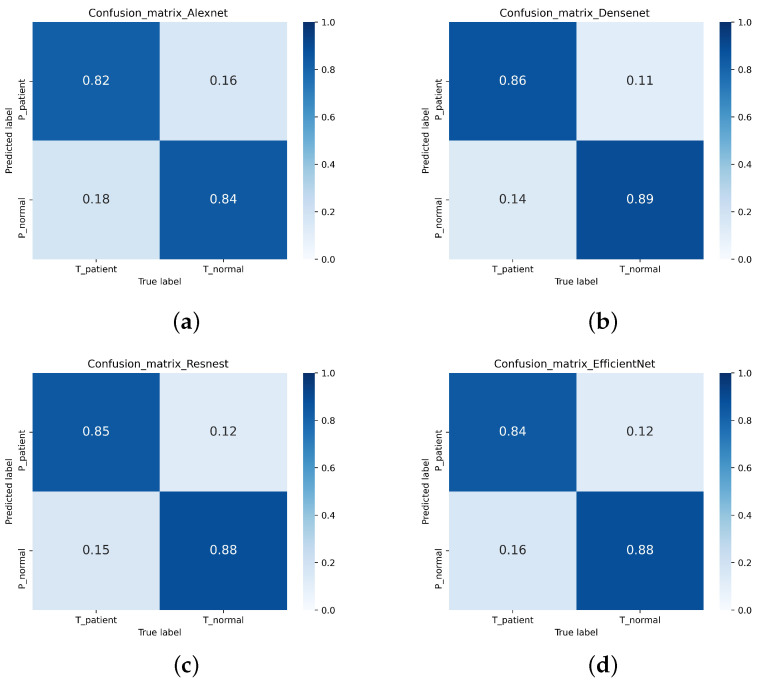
The confusion matrix of the single-mode MRI data set. (a) Alexnet (b) Densenet (c) ResNeSt (d) Efficientnet.
